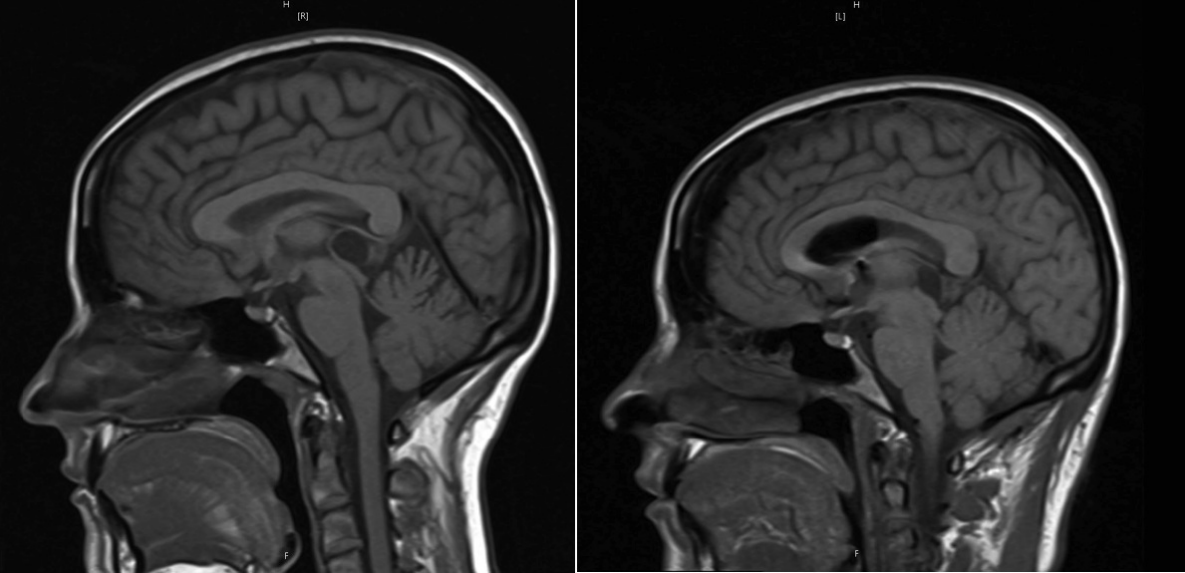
Pre- and post-operative T1-weighted magnetic resonance imaging
